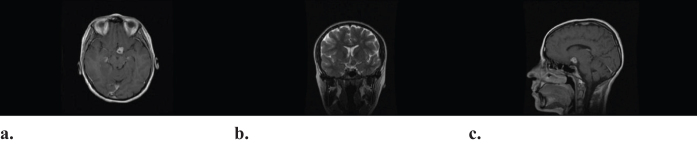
Latest MRI of the brain showing regression of tuberculomas in (a) axial T1-weighted MRI brain post-gadolinium, (b) coronal T2-weighted MRI brain post-gadolinium, and (c) sagittal T1-weighted MRI brain post-gadolinium.
